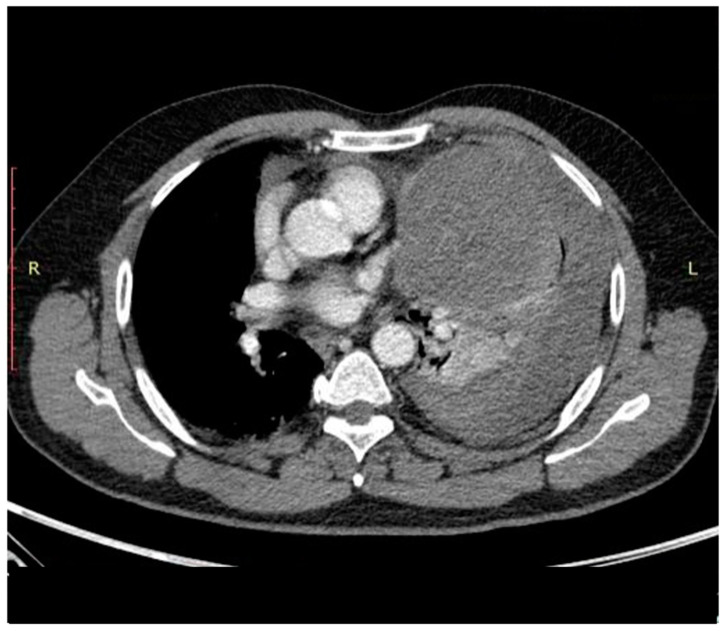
CT axial plane; Computed tomography identifies a soft-tissue mass of the left hemithorax compressing the left lung with non-specific diagnostic clues. Mediastinal involvement is best assessed by MRI.
